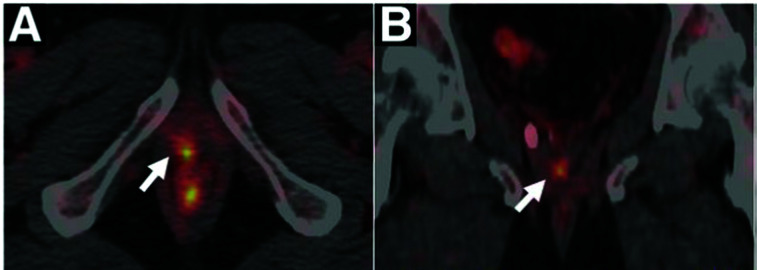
Clinical PET imaging with i.v. injected [^64^Cu]Cu–chloride to detect biochemical relapse in prostate cancer patients. The tumour is located in the pelvic region and is indicated with an arrow. This research was originally published in *J. Nucl. Med.*, Piccardo *et al.*^64^CuCl_2_ PET/CT in Prostate Cancer Relapse. *J. Nucl. Med.*, 2018, 59, 444–451. © SNMMI.^164^
